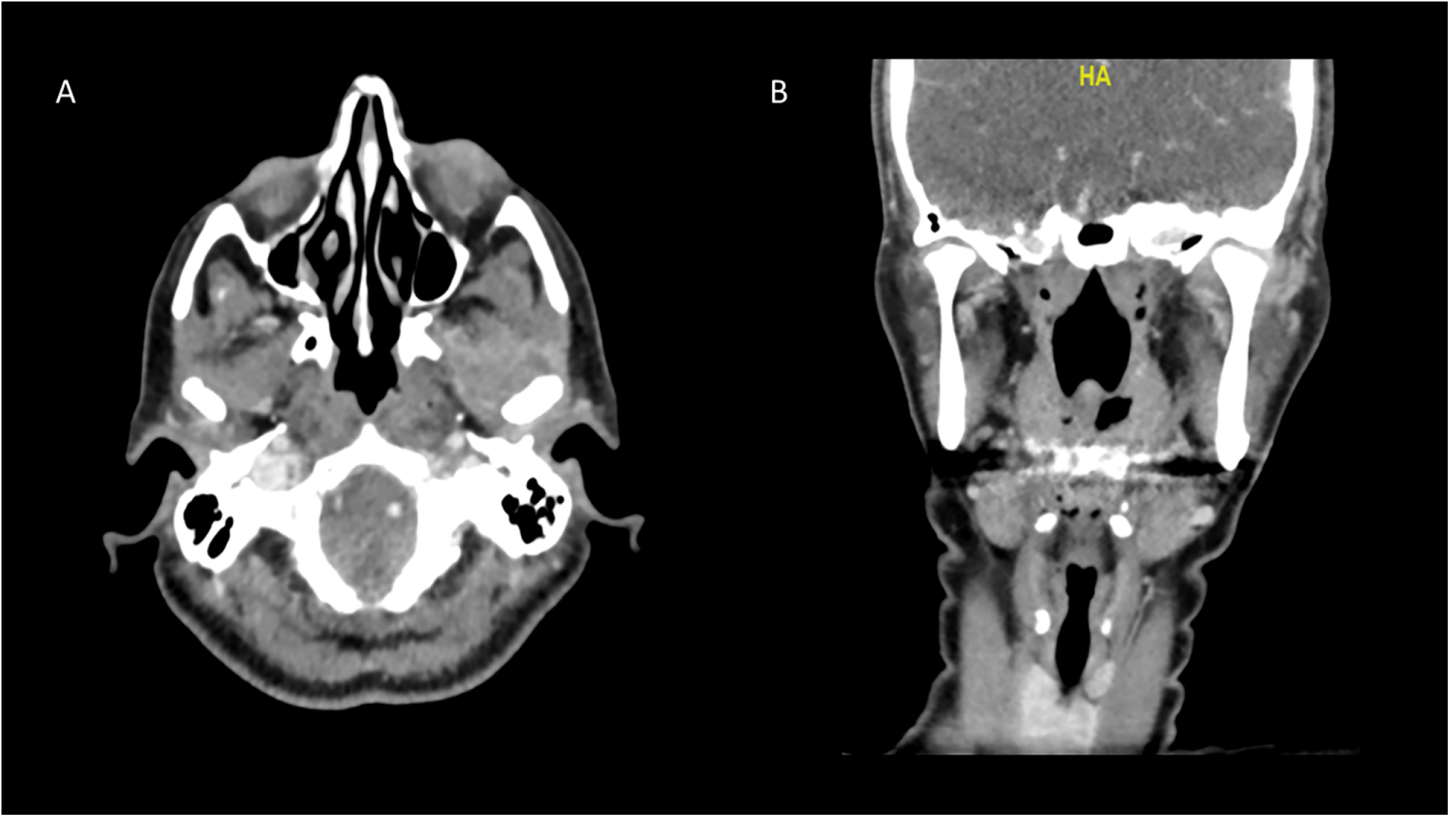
CT scan two weeks after administration of antibiotics, demonstrating almost total resolution of the collection on the left joint. This notable reduction in the collection demonstrates a positive response to treatment and indicates effective management of this severe condition. (A) Axial view, (B) coronal view.
